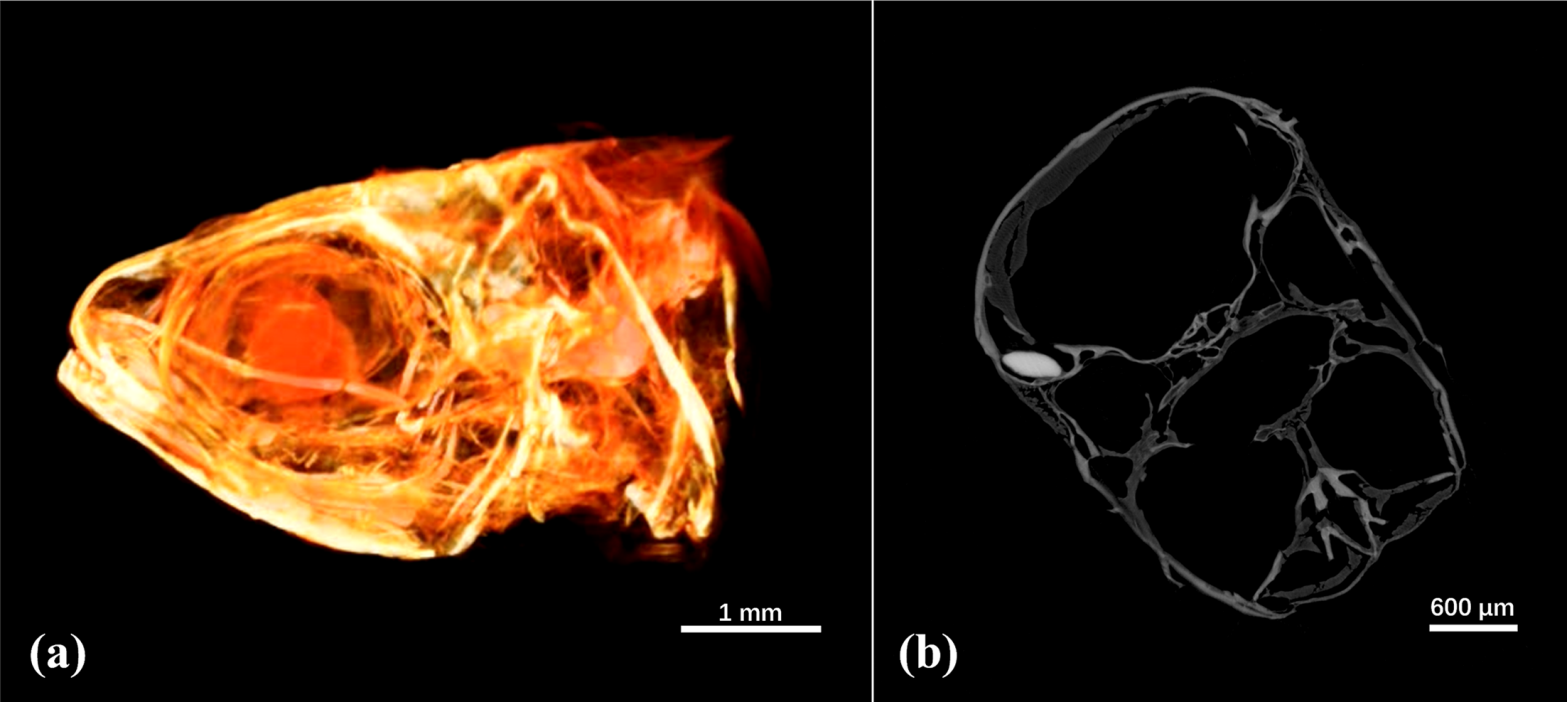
SR-CT (*a*) 3D phase-contrast imaging, and (*b*) corresponding frontal 2D reconstructed slice, of a fish head (*Poecilia reticulata*), where the different biological tissue structures can be clearly discriminated.
